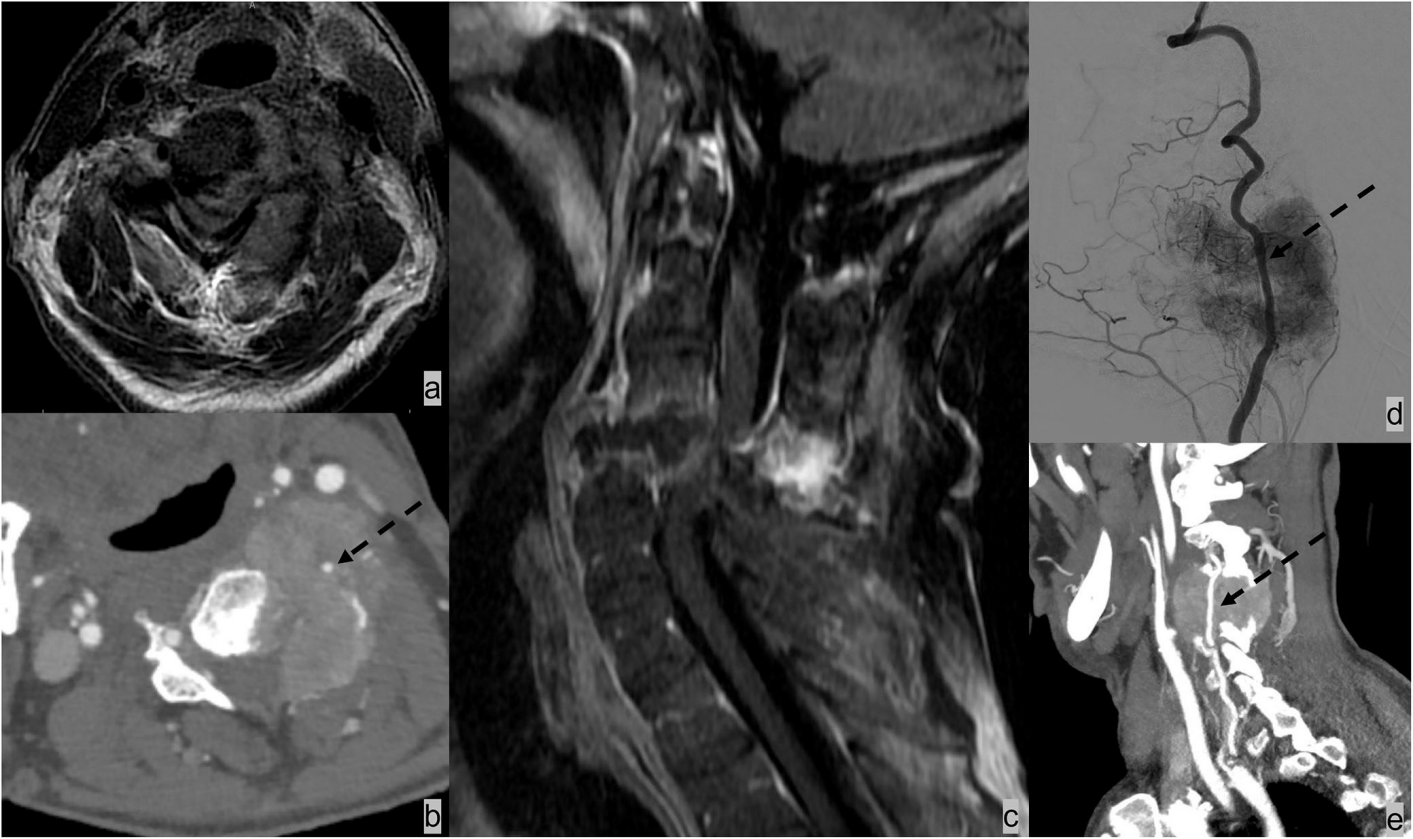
Patient had renal cell carcinoma with spinal metastases. (a–c) MRI and CT angiogram neck demonstrated osseous metastasis with soft tissue extending the ventral lateral epidural space and paraspinal space and resulting in complete effacement of the thecal sac and spinal cord compression. (b) It is further complicated by pathological fracture of C6 and posterior subluxation that resulted in mechanical instability. The metastatic lesion encases the left vertebral artery [dotted black arrow; (b,d,e)]. The patient underwent selective tumor embolization before surgical debulking and stabilization. Digital subtraction angiography demonstrates extensive tumor brush (d).
